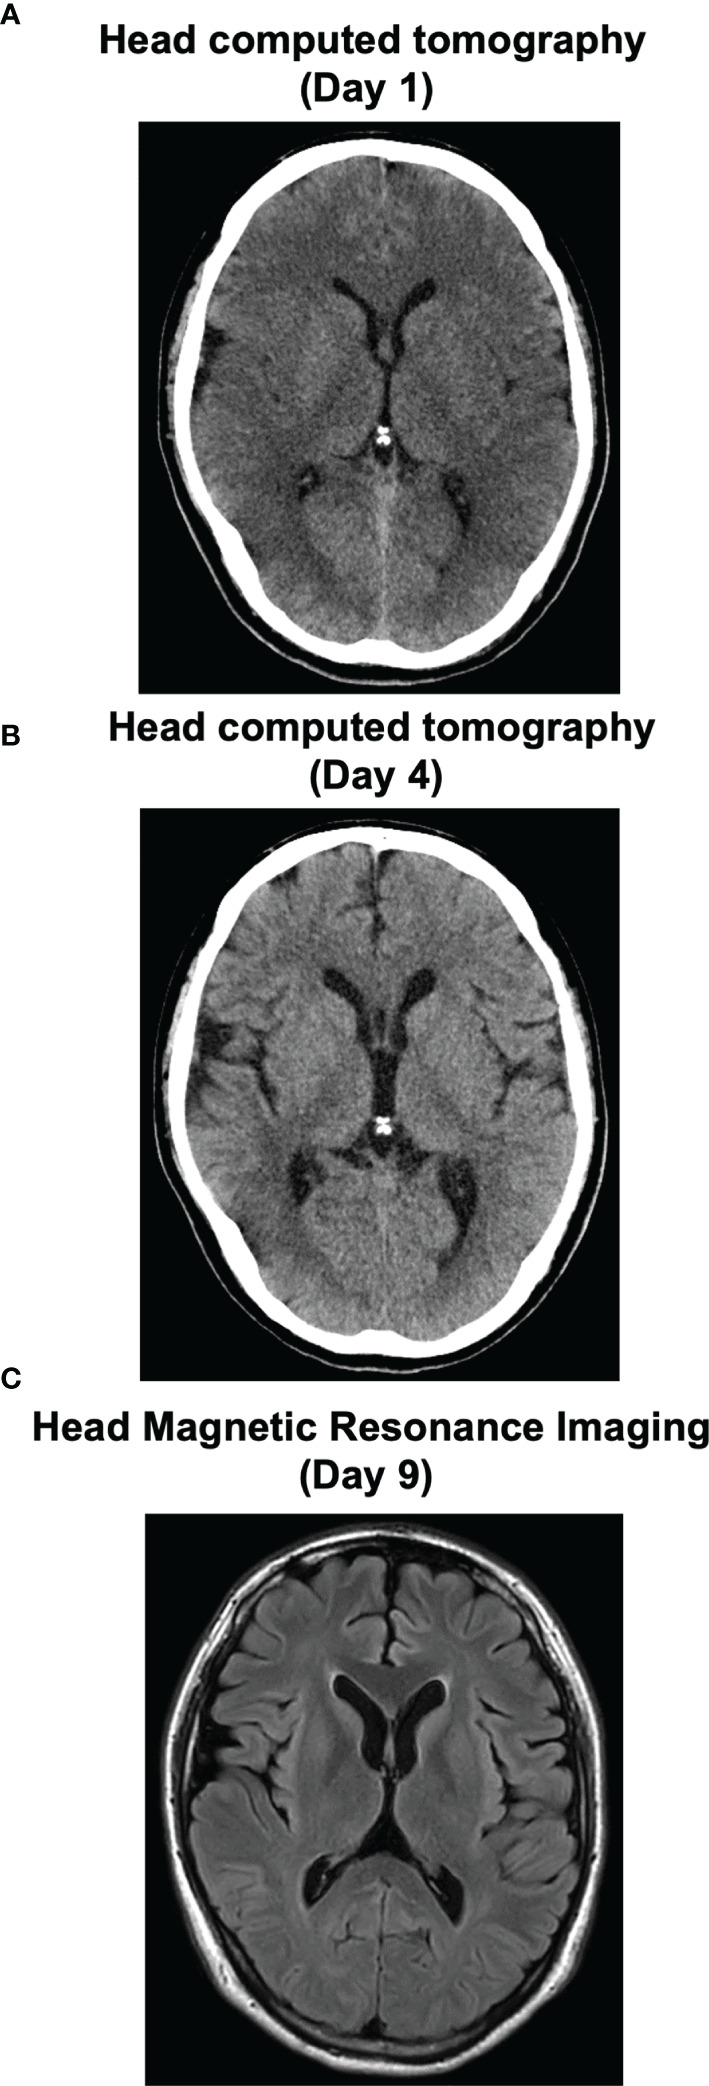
Time course of cerebral edema in this subject on the image inspection. His severe cerebral edema on admission (A) was improved at day 4 (B). At day 9, he was transferred from HCU to general ward. His head magnetic resonance imaging was normal (C).
